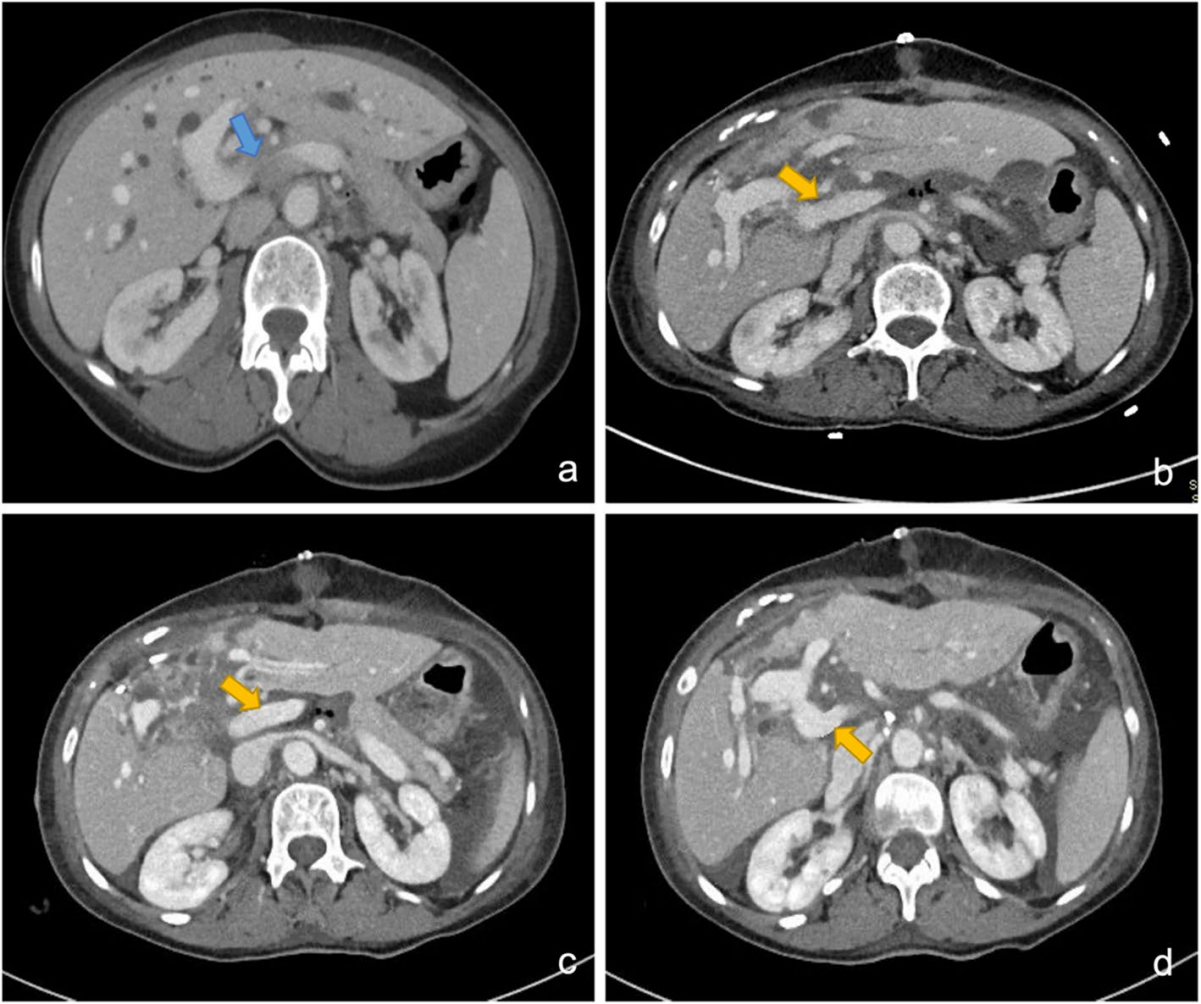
a, b, c, d Axial (a) preoperative CT section showing narrowing of portal trunk (blue arrow) above spleno-mesenteric confluence due to extensive tumor’s infiltration. Postoperative CT scans (b–d) demonstrating surgical restoration, through APG, of portal trunk patency (yellow arrows) with adequate portal inflow
